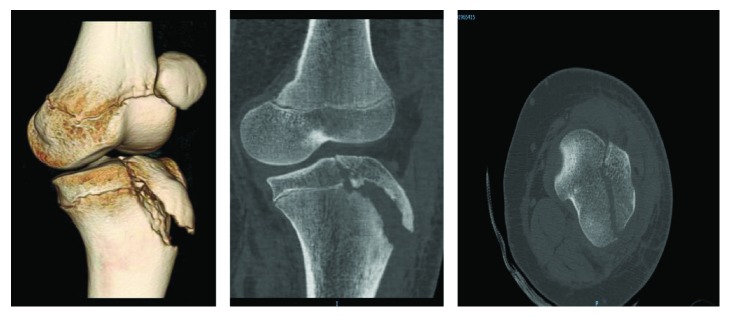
3D, sagittal, and coronal cuts of CT scan.
